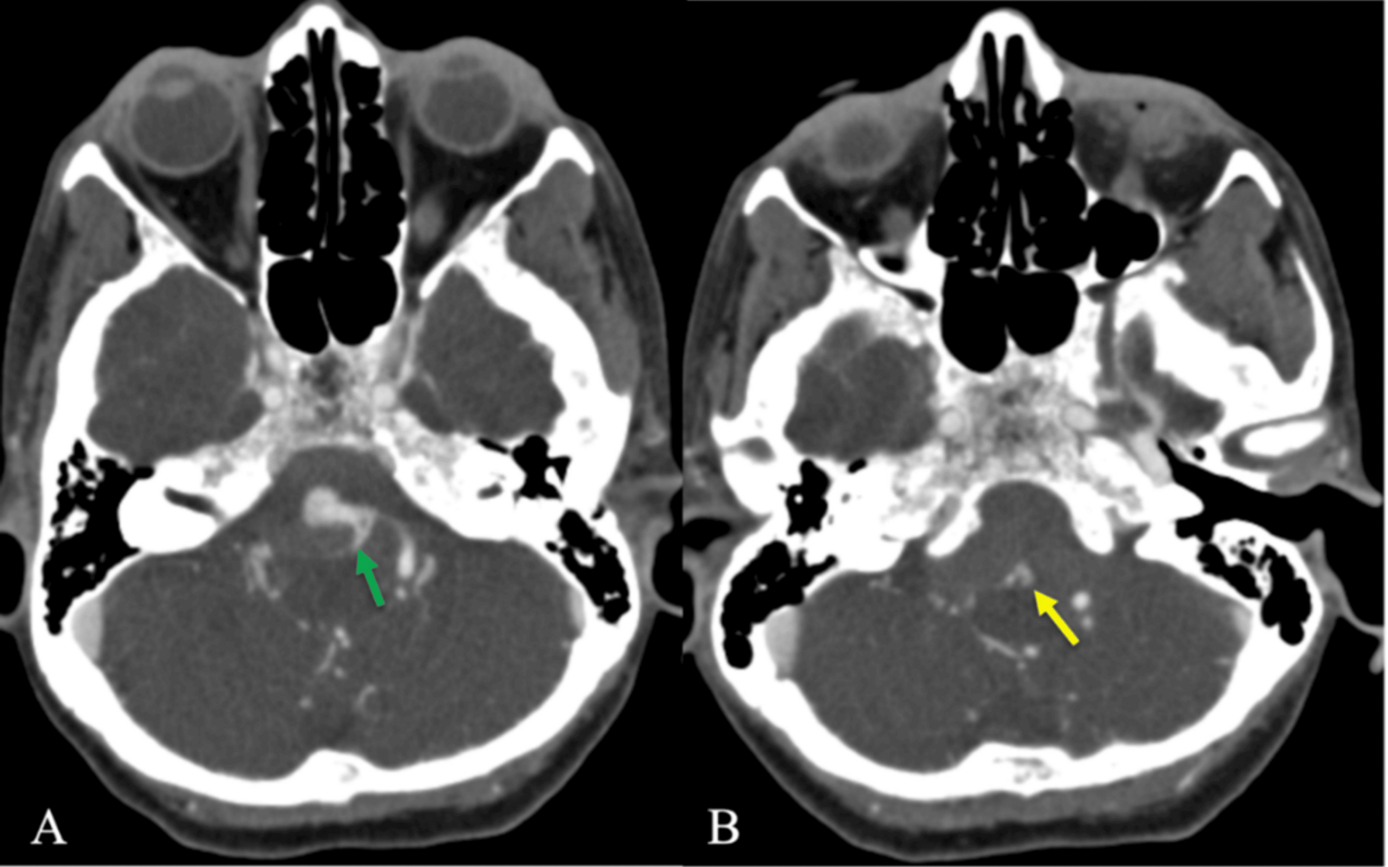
CTA demonstrating left vertebral-anterior spinal artery junction aneurysm (A) ﻿﻿﻿﻿Axial CTA head demonstrates the origin of the anterior spinal artery arising from the distal left V4 segment of the vertebral artery (green arrow). (B) ﻿﻿﻿﻿Axial CTA head at a slightly inferior level shows a small, irregular outpouching arising from the anterior spinal artery, consistent with a 2 mm saccular aneurysm (yellow arrow) CTA: computed tomography angiography
